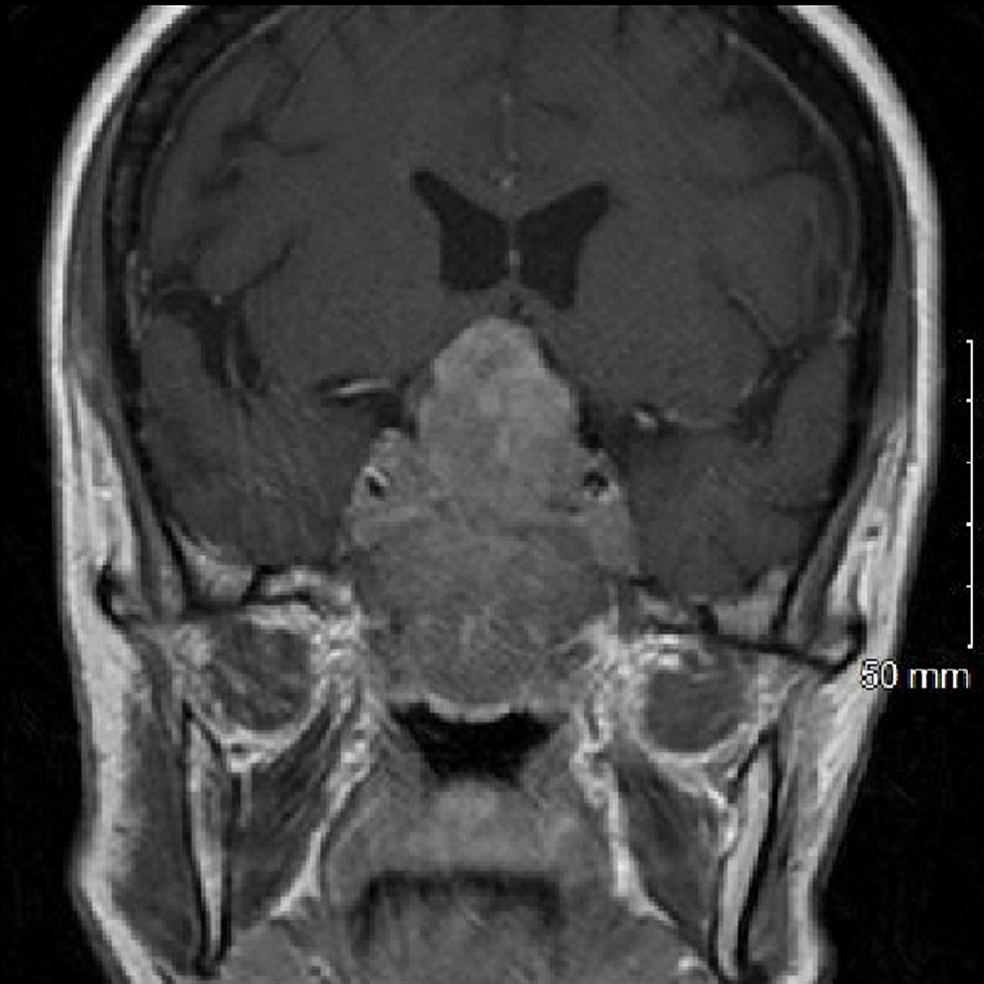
Post-contrast T1-weighted coronal reformat: a large enhancing mass has replaced the clivus and pituitary fossa, involving the posterior ethmoid region, nasopharynx and suprasellar cistern as well as the cavernous sinus bilaterally.
